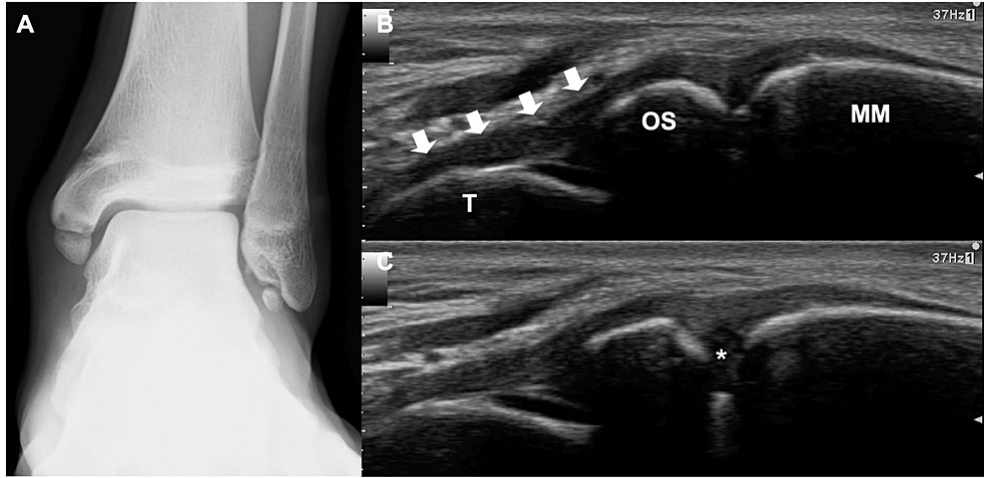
Preoperative radiograph and ultrasonographic images (A) Plain radiograph reveals a well-defined round-shaped bone beneath the medial malleolus (MM) and os subfibulare. (B) Ultrasonographic imaging reveals a superficial component of the deltoid ligament and the deep anterior tibiotalar ligament (arrows) attached to the ossicle (OS). (C) The gap (*) between the OS and the MM became wider while valgus stress was applied to the ankle. T: talar head
